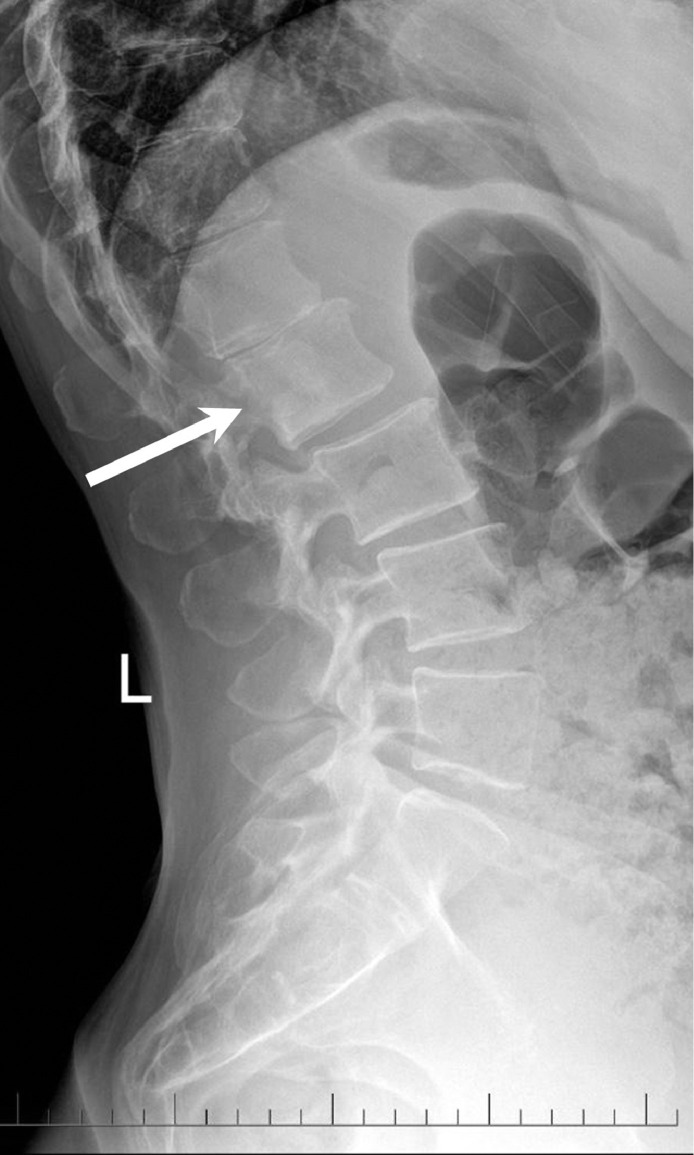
Lateral radiograph of the lumbar spine demonstrates subtle sclerosis in the posterior half of the L2 vertebral body (arrow). Intervertebral disc space narrowing is noted at T11-T12 and L1-L2. There is grade 1 retrolisthesis at L1-L2, L2-L3, and L3-L4.
